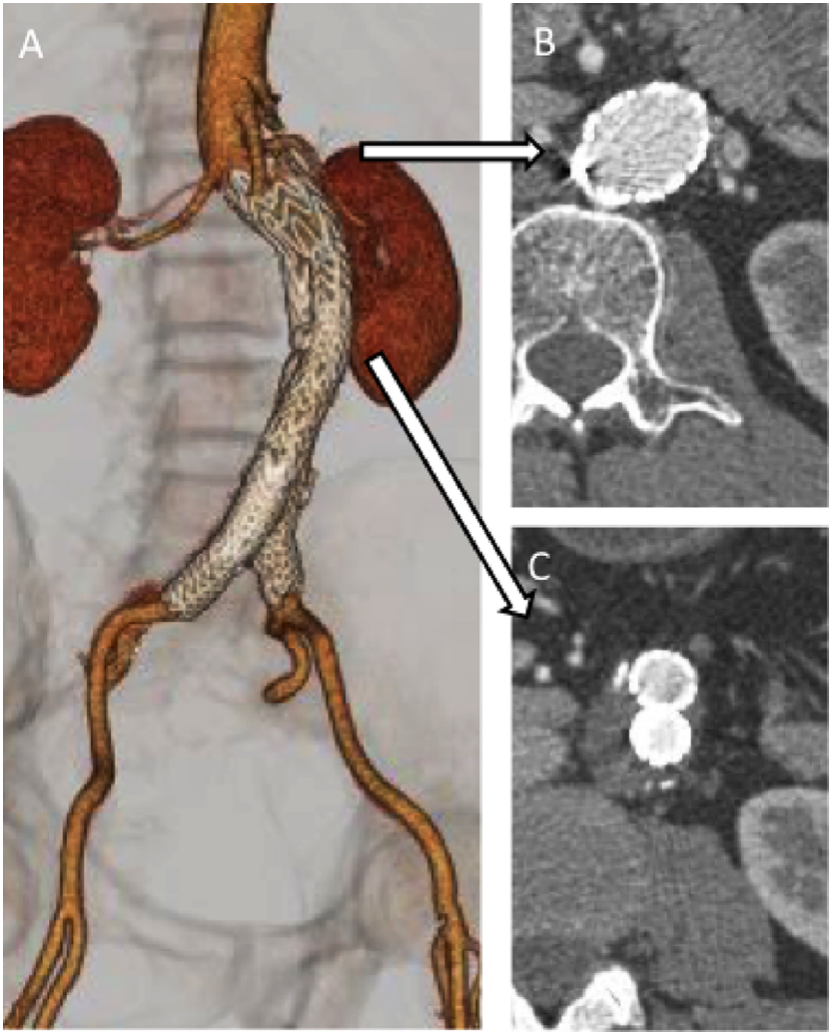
Fig. 1 (A) Aortic aneurysm has disappeared on CT scan taken before the onset of dissociation. (B), (C) The abdominal aortic aneurysm found before EVAR has almost disappeared.
